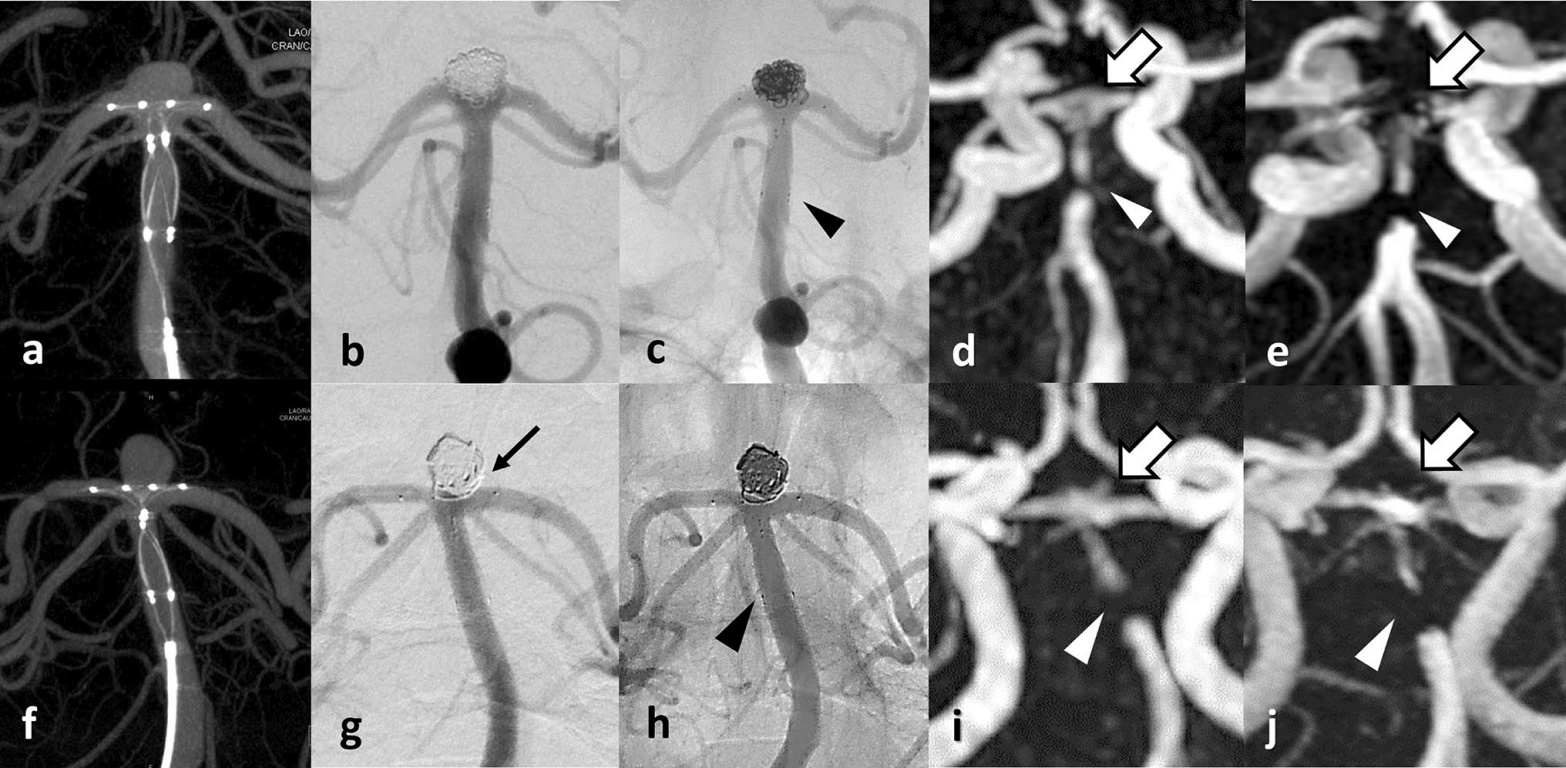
A 75-year-old female patient with an unruptured BA tip aneurysm (Case 1). a CBCT after PulseRider placement (T-shape, 10 mm, 3.5–4.5 mm). b Postoperative DSA image shows successful coil embolization with PulseRider. c Postoperative native DSA image. Black arrowhead indicates the proximal marker. d Silent MRA clearly shows the aneurysmal neck and parent artery at the bifurcation (white arrow). The segment of the proximal marker is poorly depicted (white arrowhead). e TOF-MRA failed to detect the area signal around the aneurysmal neck shown by the PulseRider device (white arrow). The segment of the proximal marker is poorly depicted (white arrowhead). A 52-year-old male patient with an unruptured BA tip aneurysm (Case 2). f CBCT after PulseRider placement (T shape, 10 mm, 3.5–4.5 mm). g Postoperative DSA image after coil embolization with PulseRider. The black arrow indicates a small neck remnant. h Postoperative native DSA image. Black arrowhead indicates the proximal marker. i Silent MRA clearly shows a small neck remnant and parent artery in the bifurcation (white arrow). The segment of the proximal marker is poorly depicted (white arrowhead). j TOF-MRA failed to detect the remnant neck clearly (white arrow). The segment of the proximal marker is poorly depicted (white arrowhead). *BA* basilar artery, *CBCT* cone-beam computed tomography, *DSA* digital subtraction angiography, *MRA* magnetic resonance angiography, *TOF* time-of-flight
